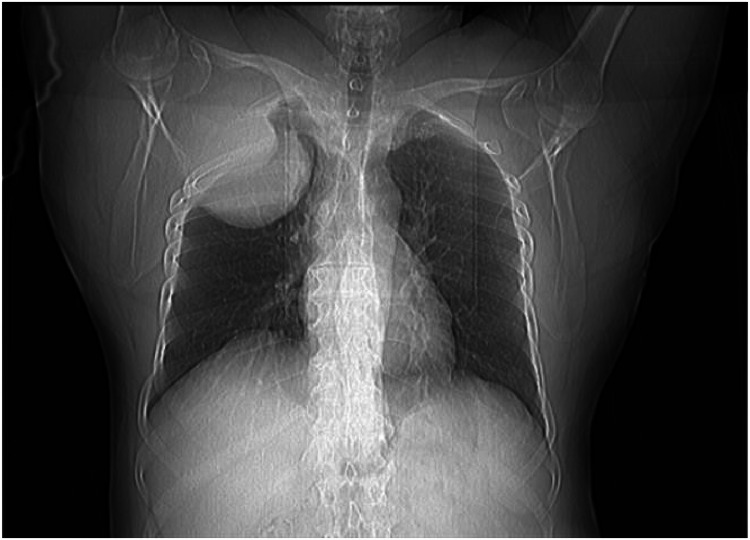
Chest radiograph showing homogenous opacity involving the right upper zone.
